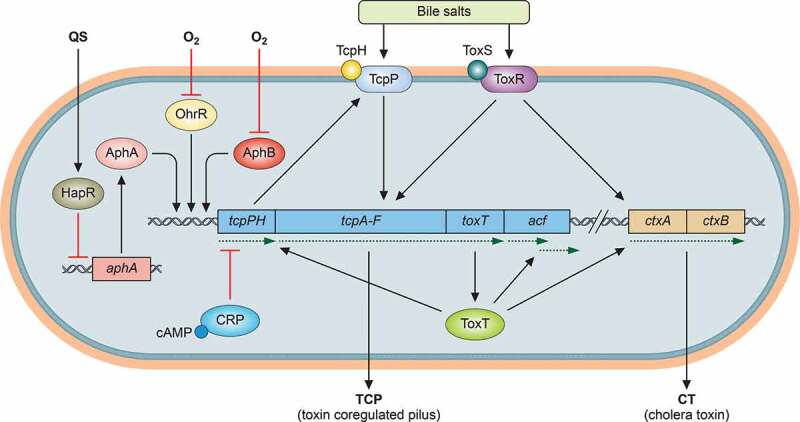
V. cholerae* virulence regulatory networks. Major transcriptional regulators and their corresponding signals are shown. In particular, the master regulator ToxT activates virulence genes which products are involved in synthesis of the key virulence determinants TCP and CT. The expression of toxT is regulated by TcpP and ToxR. QS: quorum sensing. →: activation; ┴: repression
